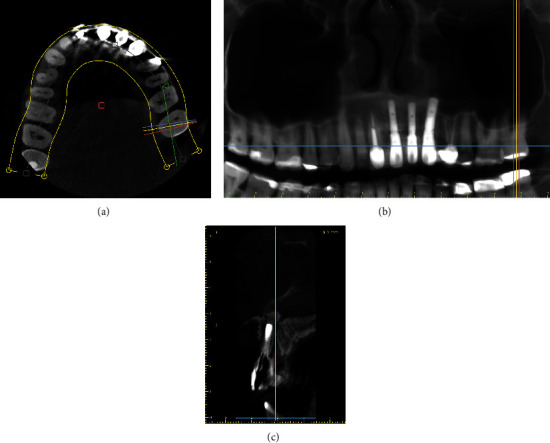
(a) Image showing sagittal section after 5 years. (b) Panoramic view showing the implant after 5 years. (c) Cone-beam computed tomography after 5-year follow-up.
